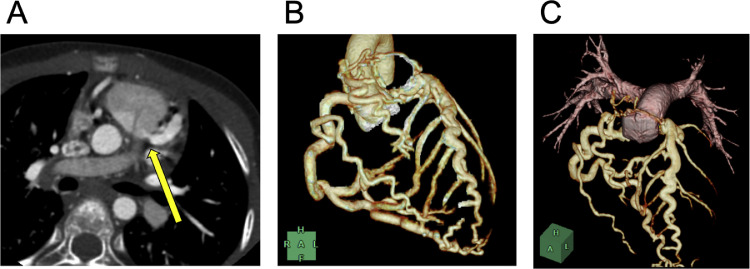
(A) Axial computed tomography showed the left coronary artery origin opened into the pulmonary artery (yellow arrows). (B-C) Multiplanar virtual reconstruction images showed dilatation and tortuosity of the RCA, showing communication between the RCA and the left anterior descending artery via the septal branch, and a collateral circulation connecting the RCA origin to the left main coronary trunk. RCA, right coronary artery.
